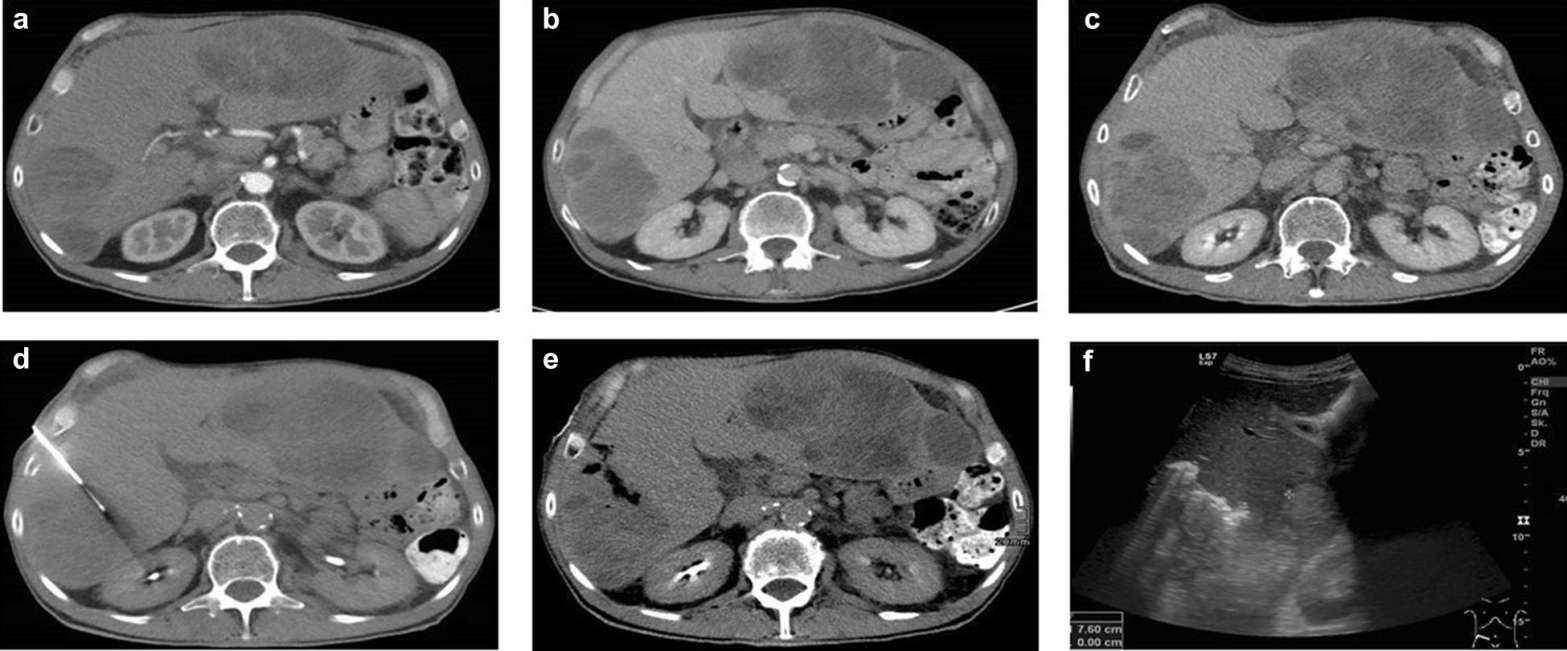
CT-guided liver biopsy and post-interventional sonography. (a) Axial slice of the pre-interventional CT. Target lesion in segment 5/6. Arterial phase. (b,c) Correlating axial slices of the lesion in late portal and delayed phase. Adequate biopsy tract length. (d) Biopsy performed with a 16-G core needle coaxial system and an 18-G cutting needle. Intralesional location. (e) Post-interventional non-enhanced CT. Percutaneous tract embolization by gelatin sponge slurry. (f) Post-interventional ultrasound. Adequate distribution of hyperechoic gelatin foam slurry. No ascites. No haematoma.
